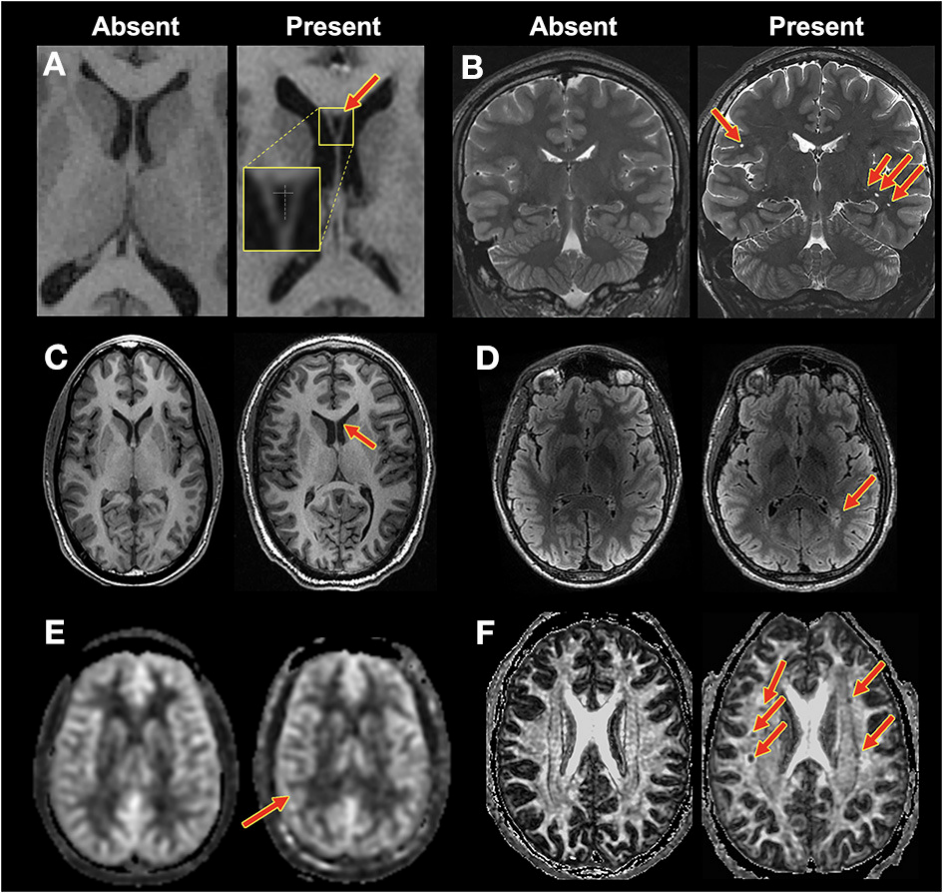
Example findings. Contrast for each image pair was as follows: (A,C): T1-weighted. (B): T2-weighted. (D): T2-FLAIR. (E): CBF. (F): DTI: FA. Red arrows indicate findings. (A) CSP: normal (1) vs. CSP (2). (B) Perivascular spaces, none (1) vs. extensive (4), and hippocampal size, normal (1) vs. mild (2). (C) Extra-axial CSF spaces: normal (1) vs. moderate (3). (D) White matter hyperintensities: none (0) vs. severe (4). (E) CBF asymmetry: normal (1) vs. possibly asymmetric (2) (F) FA holes: none (1) vs. severe (4).
